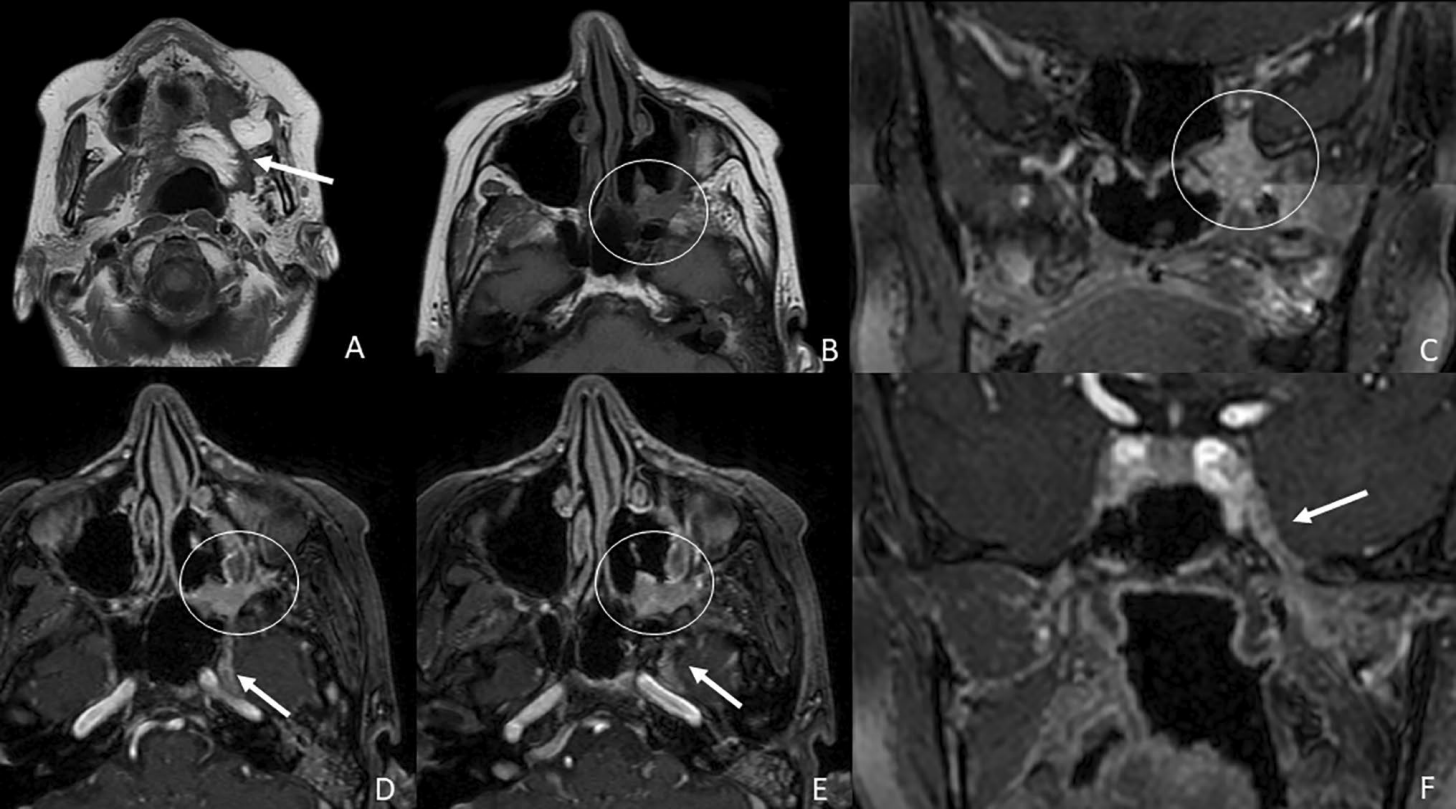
Patient with a history of oral cavity cancer treated by left hemiglossectomy with temporal flap reconstruction visible in a. (arrow) axial T1-weighted image. The axial T1-weighted image in b shows obliteration of the left pterygopalatine fossa by a pathological enhancing tissue well visible in c coronal fat-saturated 3D T1 post-contrastographic weighted images (circle). In d axial fat-saturated 3D T1 post-contrastographic weighted images it is visible a thickened enhancement extending to the V2 segment of the left trigeminal nerve (arrow) and in e axial and f coronal fat-saturated 3D T1 post-contrastographic weighted images a thickened enhancement of the ipsilateral V3 segment (arrow). These findings are consistent with perineural spread
